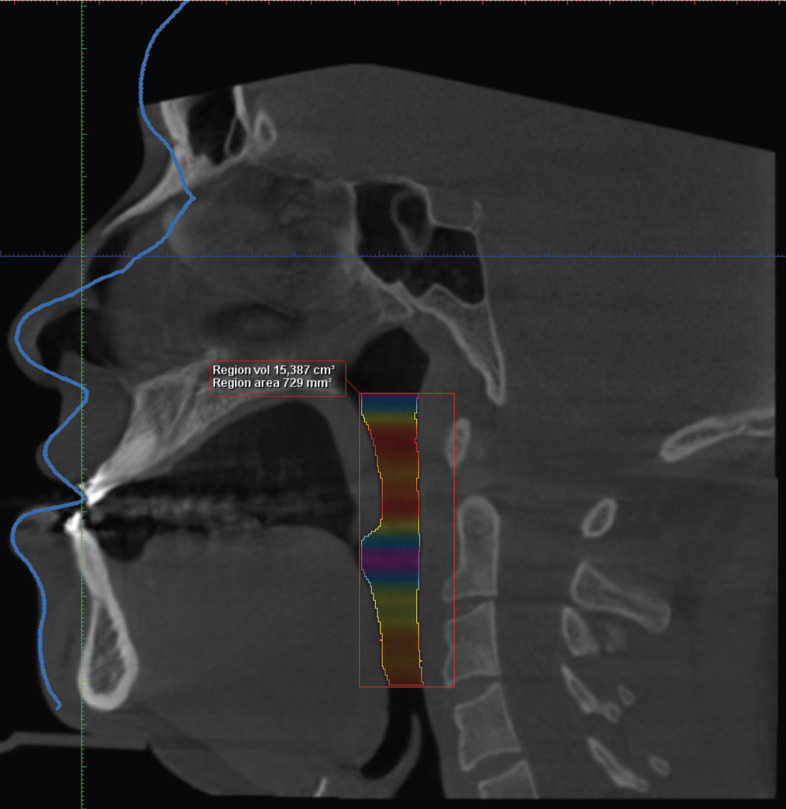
Referential image of CBCT in sagittal view with total volume of the UA recognized by Romexis software.
